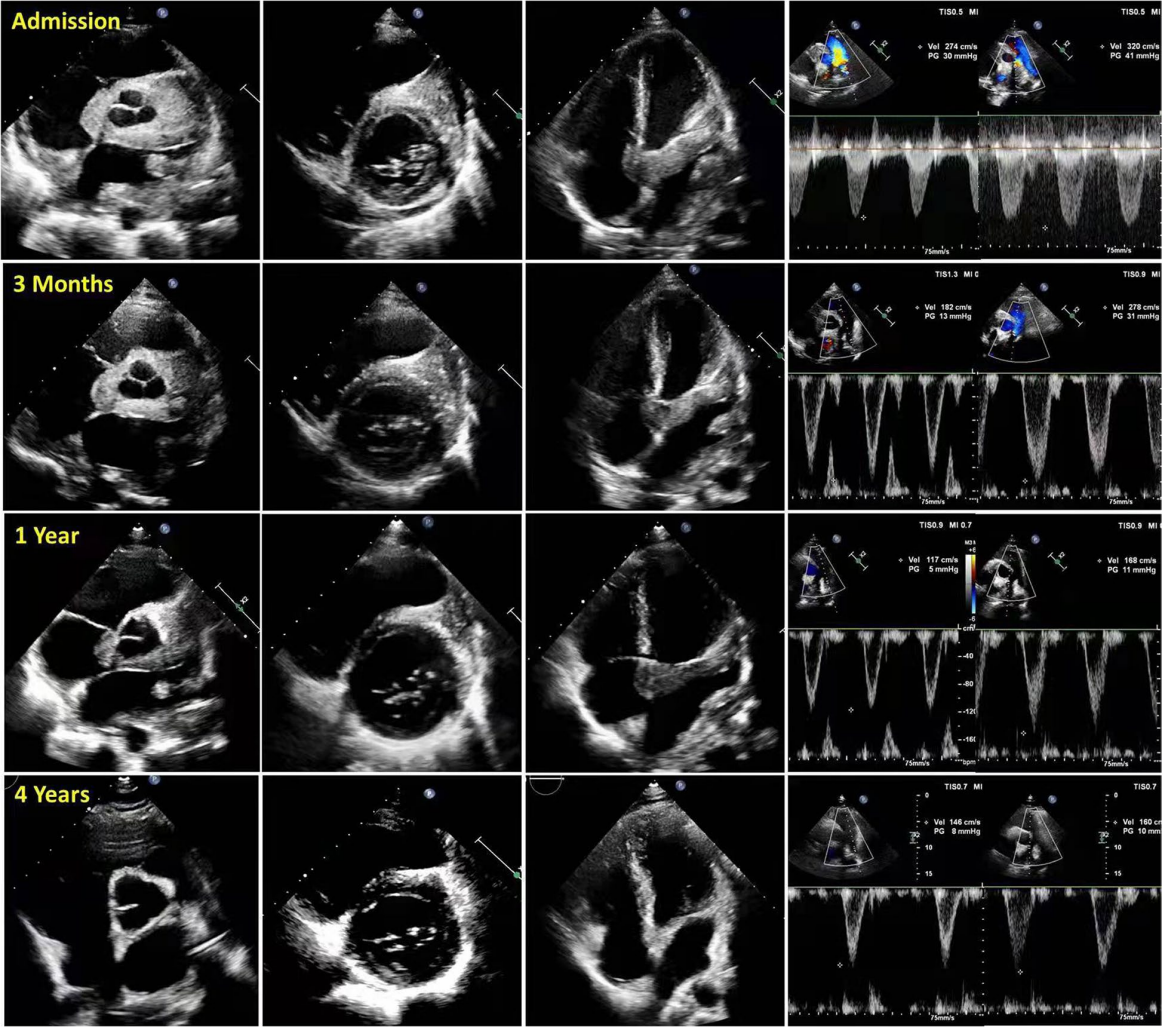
Echocardiography at admission and at different times after treatment showed the area of the lesion became significantly smaller and the blood flow velocity of the pulmonary artery branch returned to normal
